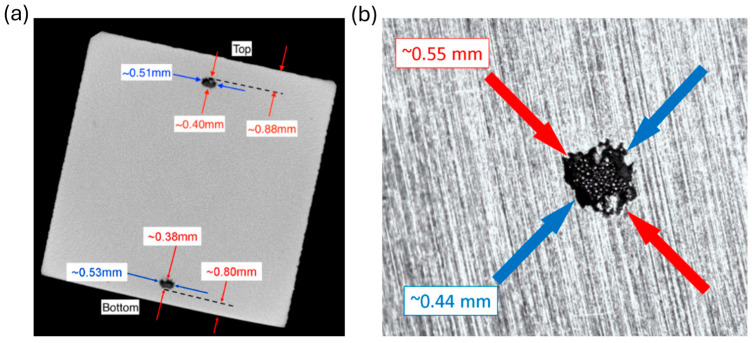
Images of physical flaws. Radiograph image in (a) shows the sub-surface cavities found in Samples C. Image (b) shows an exposed sub-surface void from Sample A.
